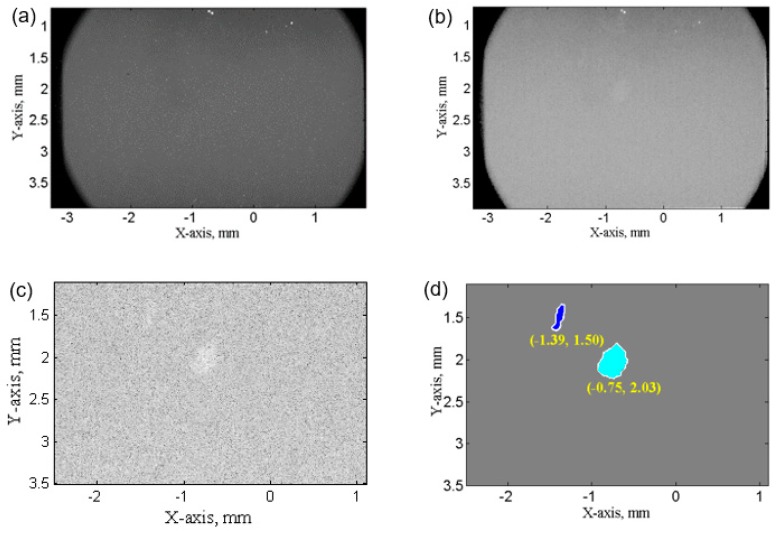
A typical X-ray raw image taken in the SCR region. (a) before image processing; (b) after flat-field correction; (c) cropped X-ray image after normalization by image taken without UST, and contrast enhancement; (d) The final binary image, which was used to extract the position and size of ultrasonic bubbles.
